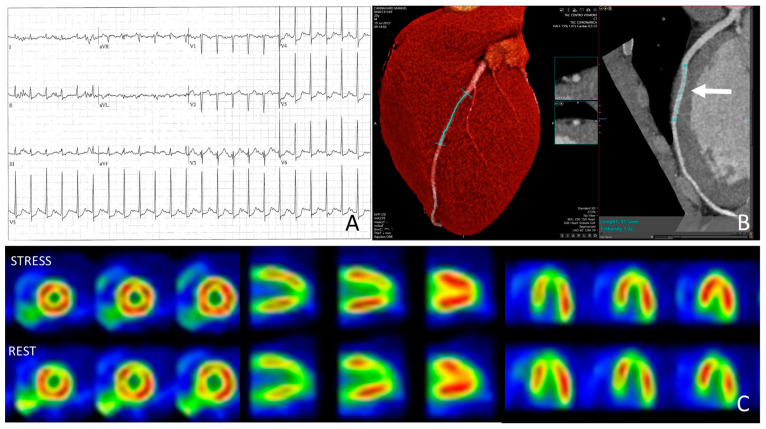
Representative example of an asymptomatic athlete with ST-segment depression performed for preparticipation screening (A). Coronary computed tomography revealed myocardial bridging of the left anterior descending artery (B). However, stress-rest myocardial SPECT showed no inducible myocardial ischemia (C).
